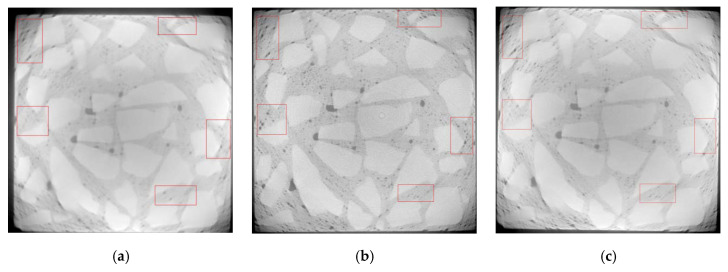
CT images of D4 under different dry and wet cycles of sulfate attack: (a) 0 dry–wet cycle; (b) 40 dry–wet cycles; (c) 80 dry–wet cycles.
